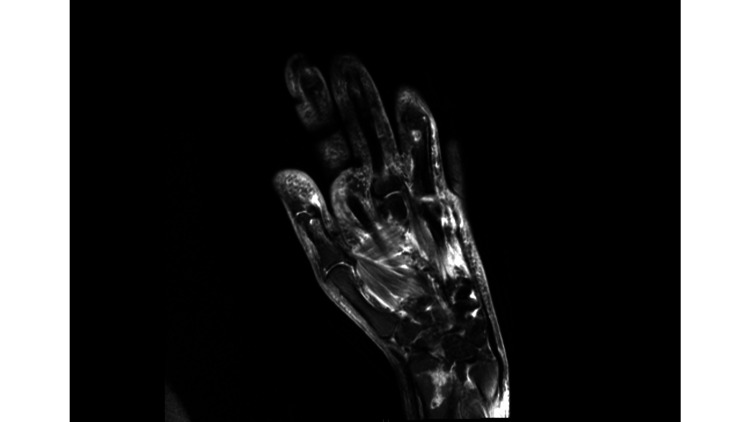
MRI of the right wrist without contrast Seen in this image is a comminuted intra-articular distal radial fracture with surrounding marrow edema along the fracture margins. The intramuscular signal is grossly normal without evidence of fluid collection, which suggests that a soft-tissue infection or hematoma is unlikely to be the cause of the patient's persistent wrist swelling.
